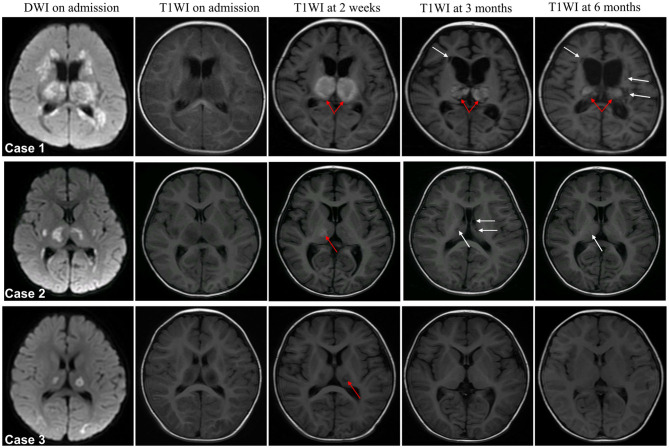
Representative dynamic MRI images of survival ANE patients with different degrees of neurological sequelae. Case 1 with severe, case 2 with moderate and case 3 with mild neurological sequelae. Arrow in red indicates hemorrhage and white indicates cavitation.
